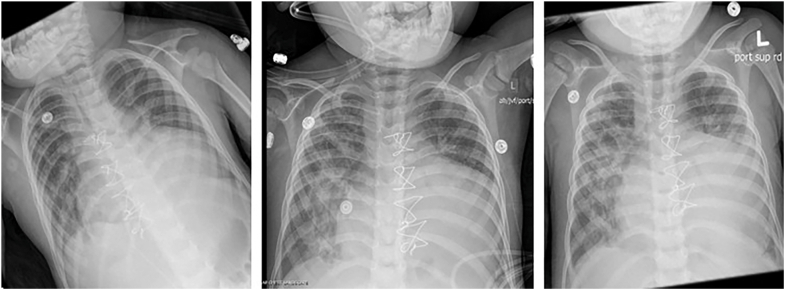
Findings on chest radiography from days 2 to 4 of admission demonstrate progressive worsening of bilateral opacities contributing to elevated pulmonary pressures and pulmonary hypertensive crisis.
